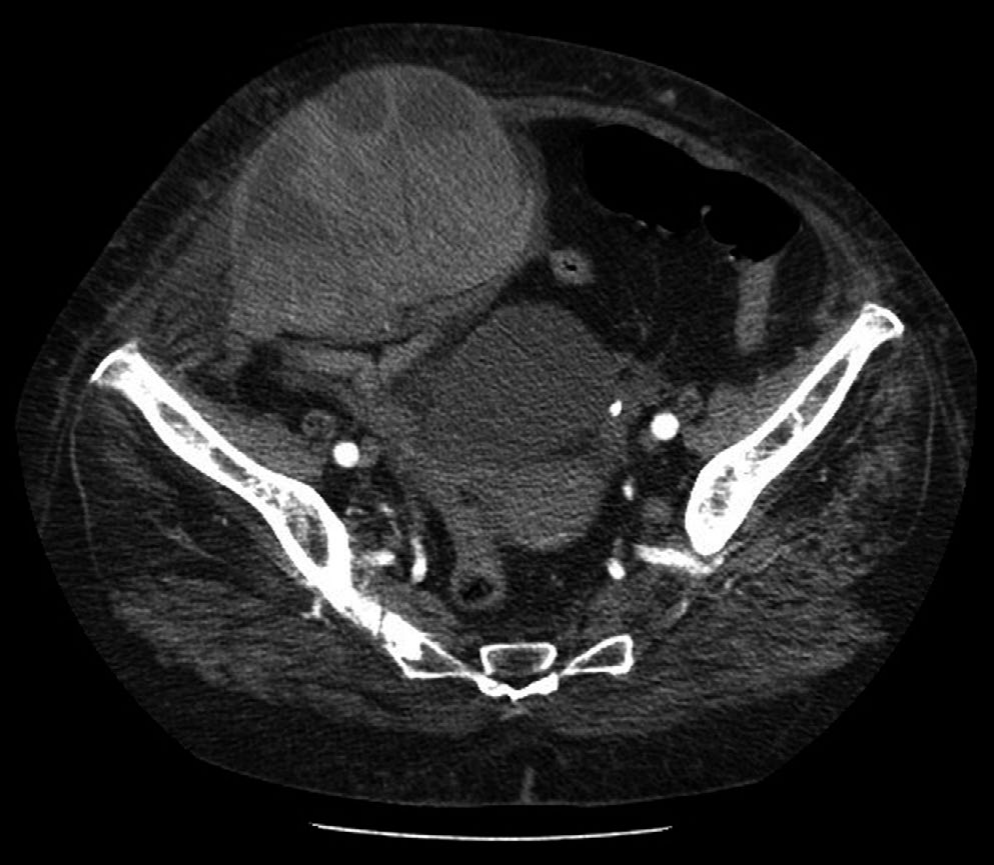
Case 5: CT scan of the abdomen and pelvis revealing a right rectus sheath hematoma. Large expansive oval formation depending on the abdominal wall of the right hemi‐abdomen, very markedly heterogeneous, with areas of greater and lesser density, compatible with a hematoma. After contrast injection (arterial phase), denser foci are observed, indicating active bleeding
